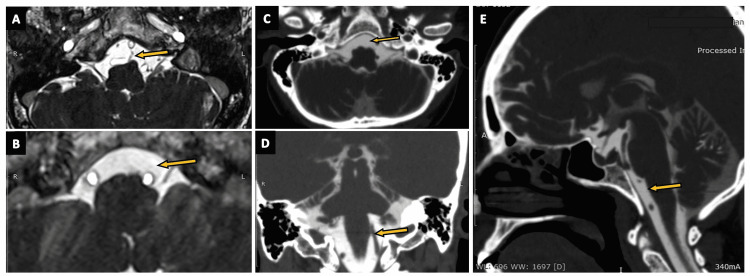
The medullary cistern. (A) The medullary cistern (arrow) in the axial plane of the three-dimensional (3D) constructive interference in steady state (CISS) MRI sequence. (B) A zoomed-in portion of an axial plane of the 3D-CISS MRI sequence showing the medullary cistern (arrow). (C), (D), and (E) show the medullary cistern (arrows) in the axial, coronal, and sagittal sections of CT cisternography, respectively, in a case of suspected CSF rhinorrhea.
